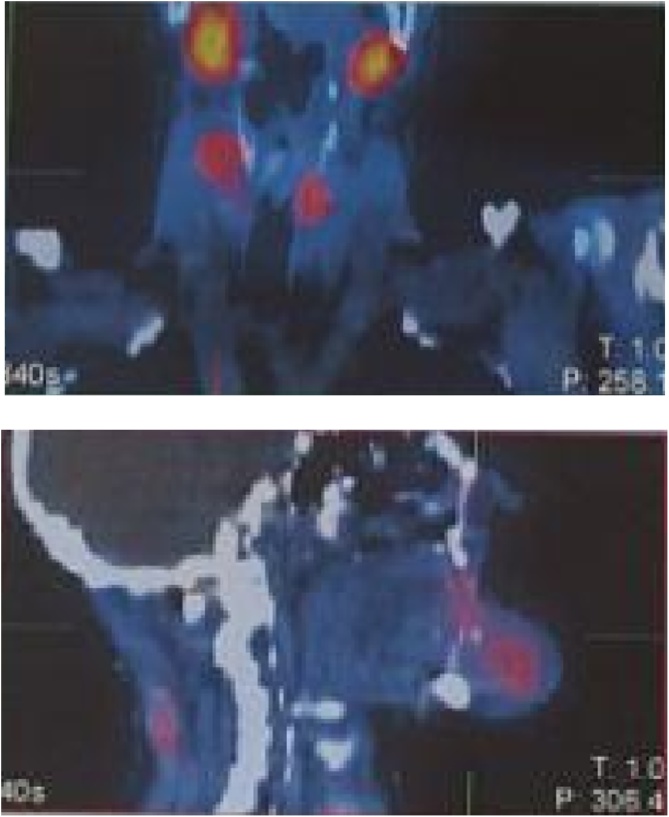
SPECT-CT parathyroid scan. bilaretal parathyroid adenomes and parathyroid hyperplasia.
